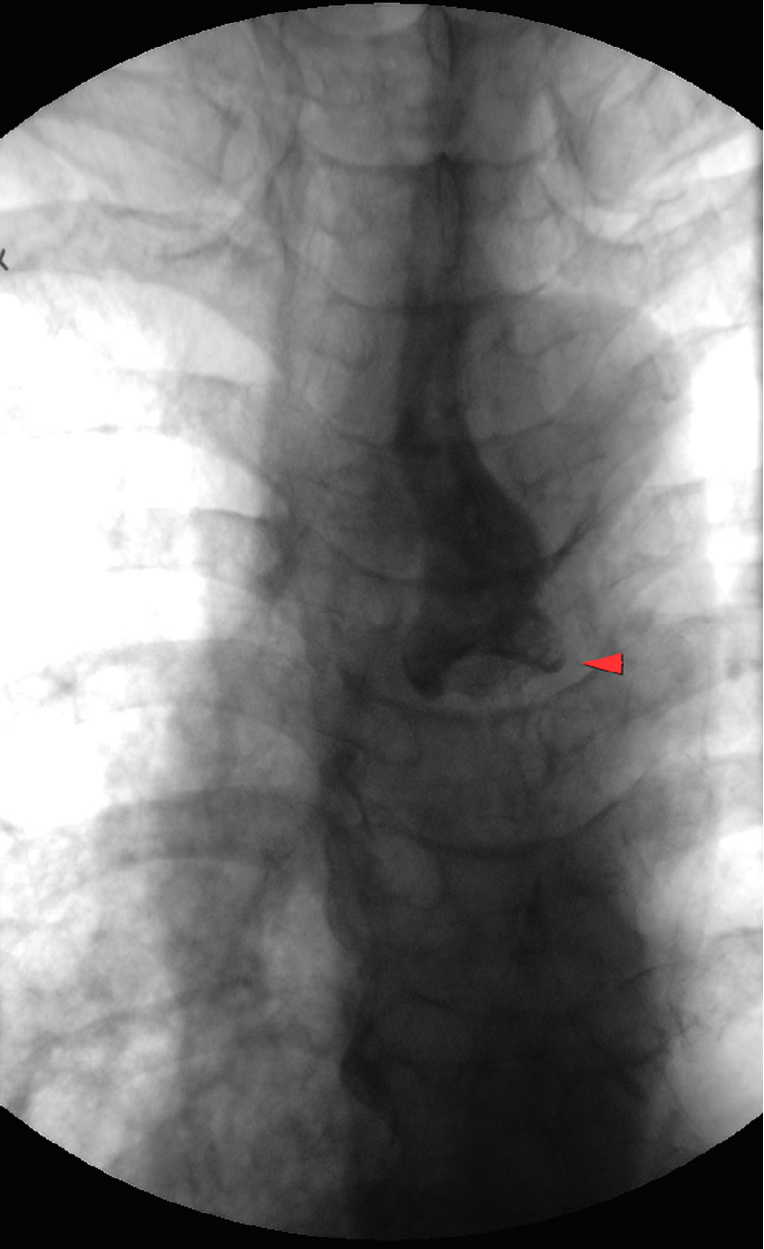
Anterior chest radiograph taken after videofluoroscopic swallowing study (red arrow head pointing to the pooling of contrast material above the mid‐thoracic esophagus).
